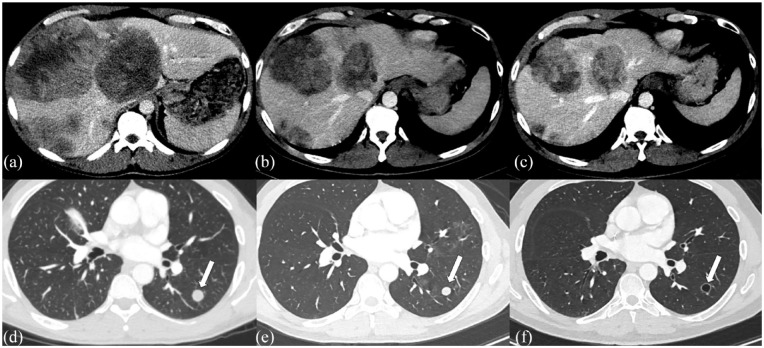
CT scan of chest, abdomen, and pelvis. Hepatic and pulmonary metastatic lesions response to treatment. (a) Multiple liver masses at the time of diagnosis. (b) Liver lesions following four cycles of etoposide + ifosfamide + cisplatin (VIP regimen). (c) Liver lesions following two cycles of FLOT (5-FU, leucovorin, oxaliplatin, docetaxel) plus pembrolizumab. (d) Multiple left lower lobe pulmonary nodules (largest indicated with arrow) at the time of diagnosis. (e) Lung nodules following four cycles of VIP regimen. (f) Lung nodules following two cycles of FLOT plus pembrolizumab.
